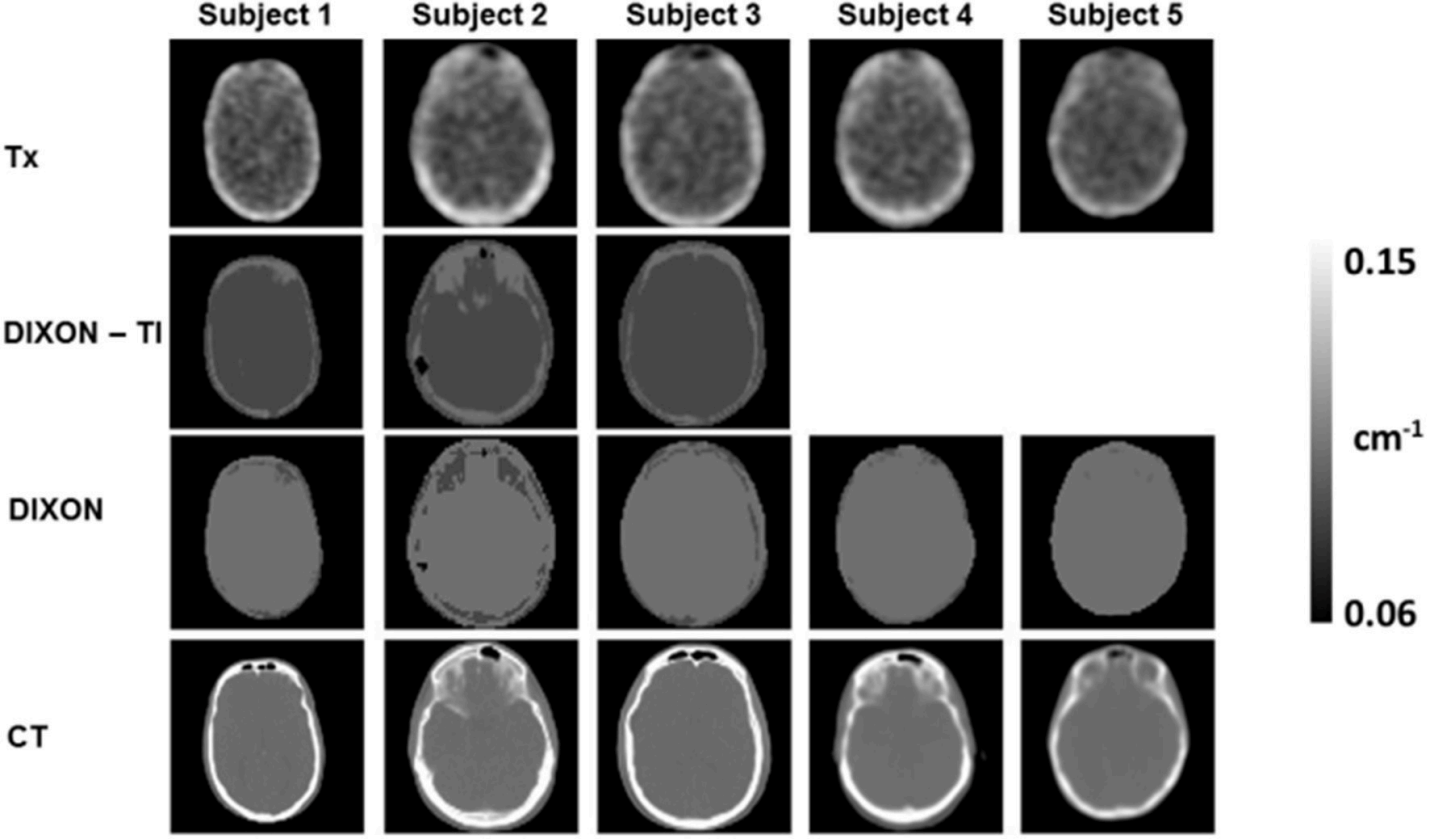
Measured and derived ATN maps obtained for the five subjects. Tissue-inversion (TI) was observed for 3/5 DIXON-ATN maps (row 2). Affected ATN maps were corrected using equation 1, which resulted in new ATN maps as displayed in row 3 (DIXON). A subsequent low-dose CT was obtained for all subjects in a Siemens TPTV system (row 4, CT).
